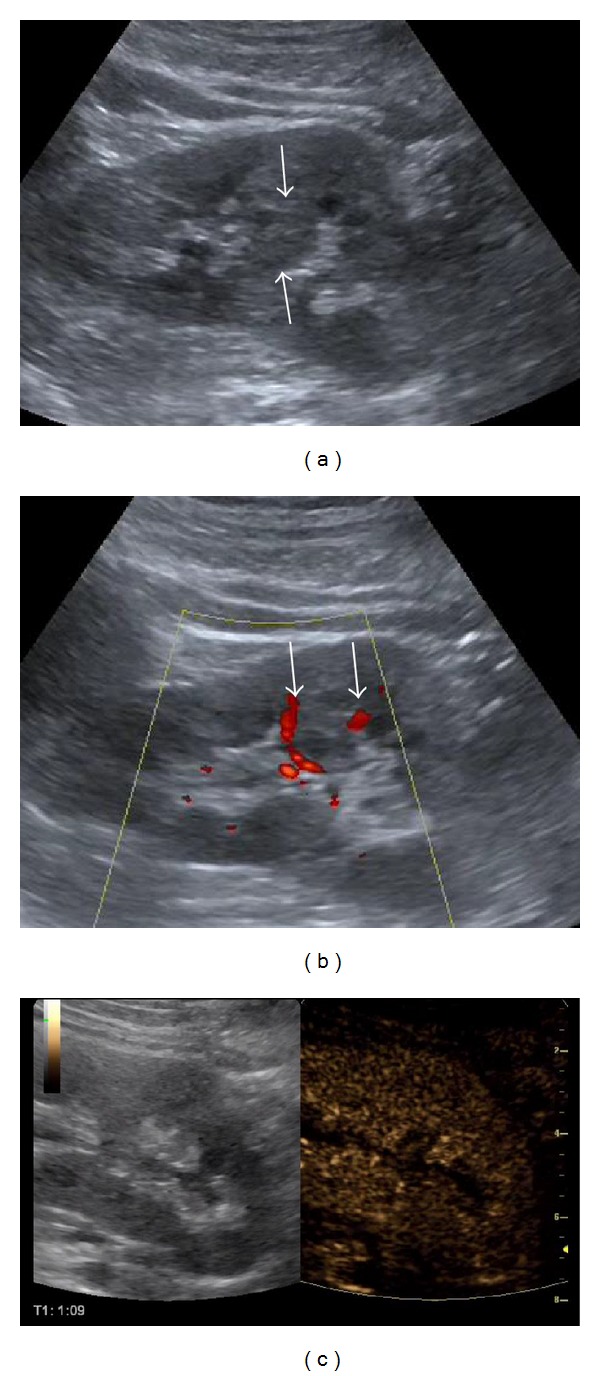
Kidney pseudotumour: a solid isoechoic area is noted in the middle of the left kidney on B-mode US (arrows in (a)), seeming to displace blood vessels on Colour Doppler (arrows in (b)). After SonoVue injection (c), this area enhances in the same way as the rest of the renal parenchyma, suggestive of a pseudotumour of no clinical significance.
